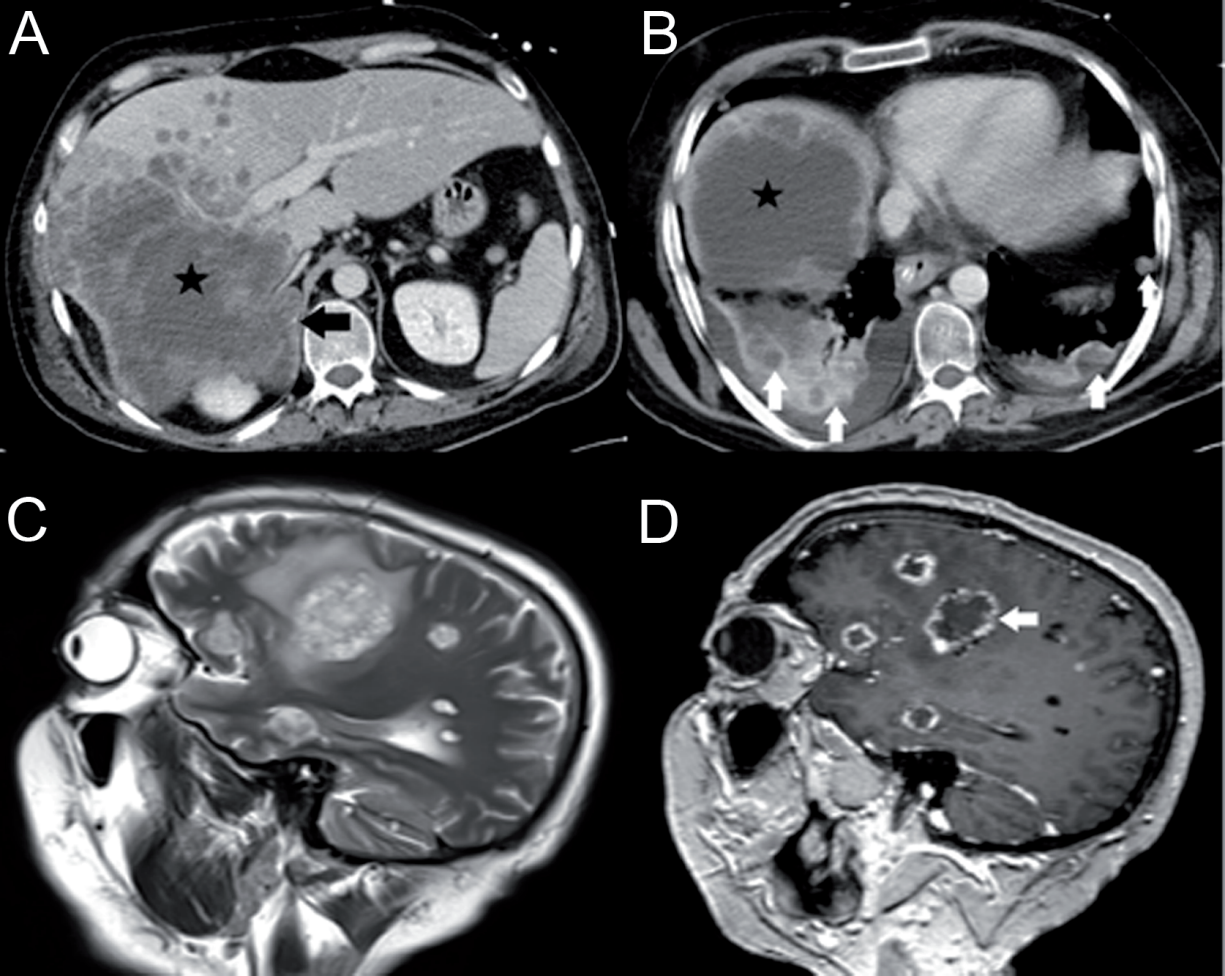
Axial contrast-enhanced computed tomography (CT) imaged at the level of the liver (A) and lung basis (B) shows alveolar echinococcosis lesion in the liver (black stars in A and B) and lung (white arrows in B). In addition to the liver lesion, sagittal T2-weighted magnetic resonance (MR) image (C) and sagittal T1-weighted contrast-enhanced image (D) show multiple alveolar echinococcosis metastases in the brain. The lesion in the liver appears to invade the right adrenal gland with local invasion (black arrow in A). Note that metastases observed in the brain show peripheral contrast enhancement (white arrow in D) and vasogenic edema effect is observed around the lesions on sagittal T2-weighted MR images.
